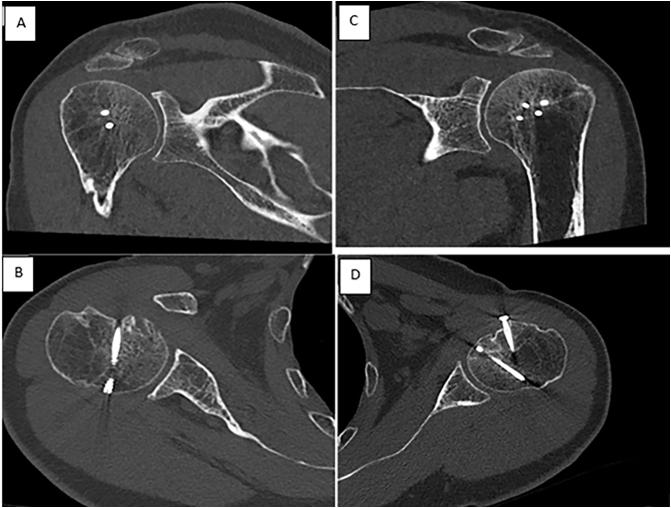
8 months follow-up CT scan. A, B: Right shoulder; C, D: left shoulder.
